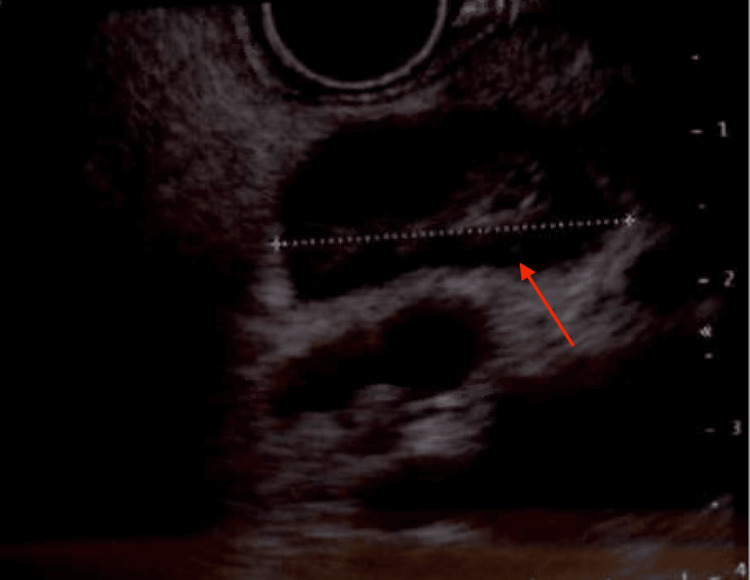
EUS showing an enlarged 2.3 cm perigastric lymph node. EUS, endoscopic ultrasound
